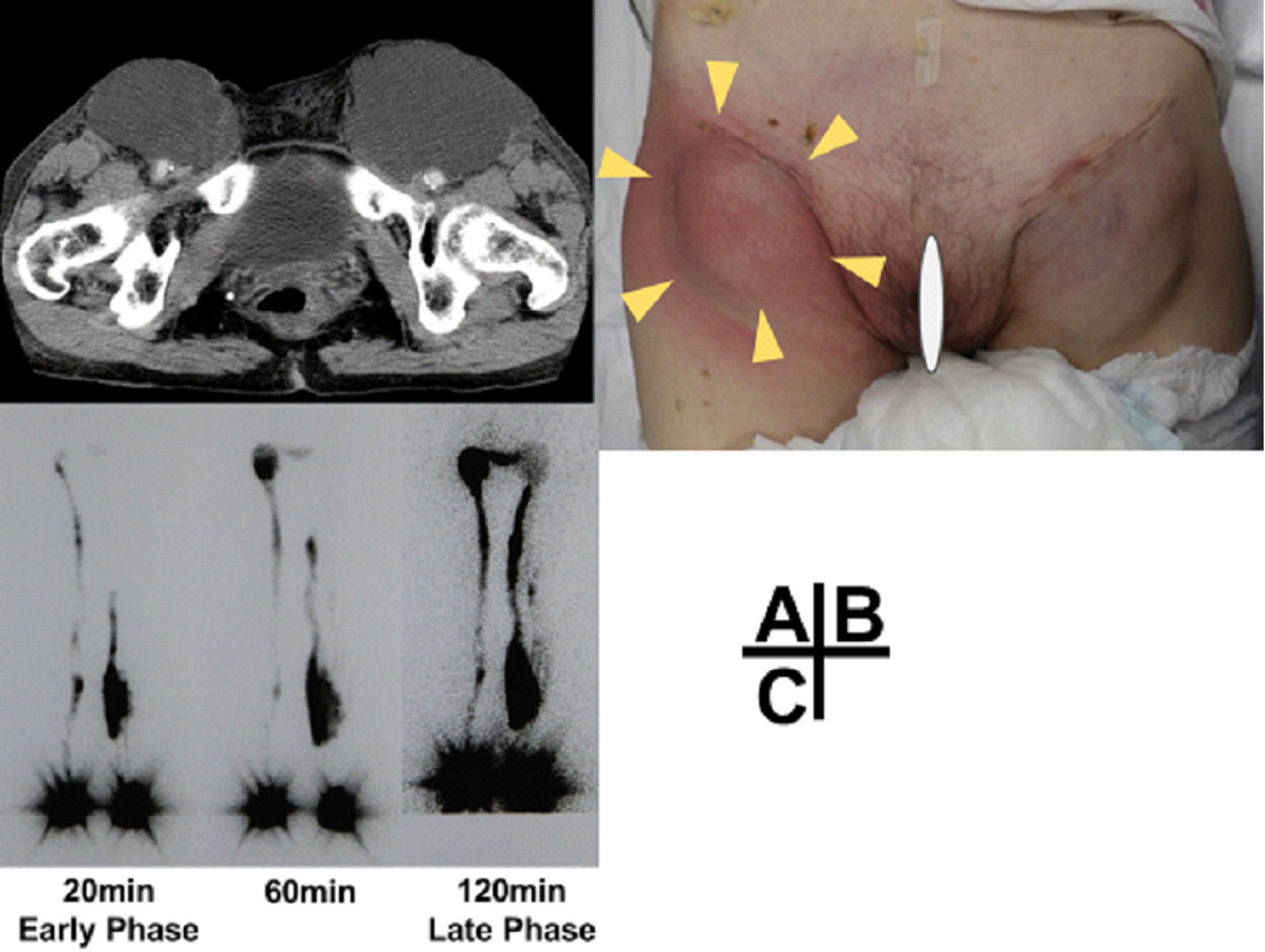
(a) Lymphocysts formed in the bilateral inguinal region found on computed tomography. (b) External appearance of lymphocysts formed in the bilateral inguinal region with the right lymphocyst showing signs of infection (pointers). (c) Lymphoscintigraphy results: Dermal backflow can be observed in the left lower leg during the early phase, while Radio Isotope leakage to bilateral inguinal lymphocysts and stasis in the collecting lymphatic vessel can be seen in the images taken during the late phase.
